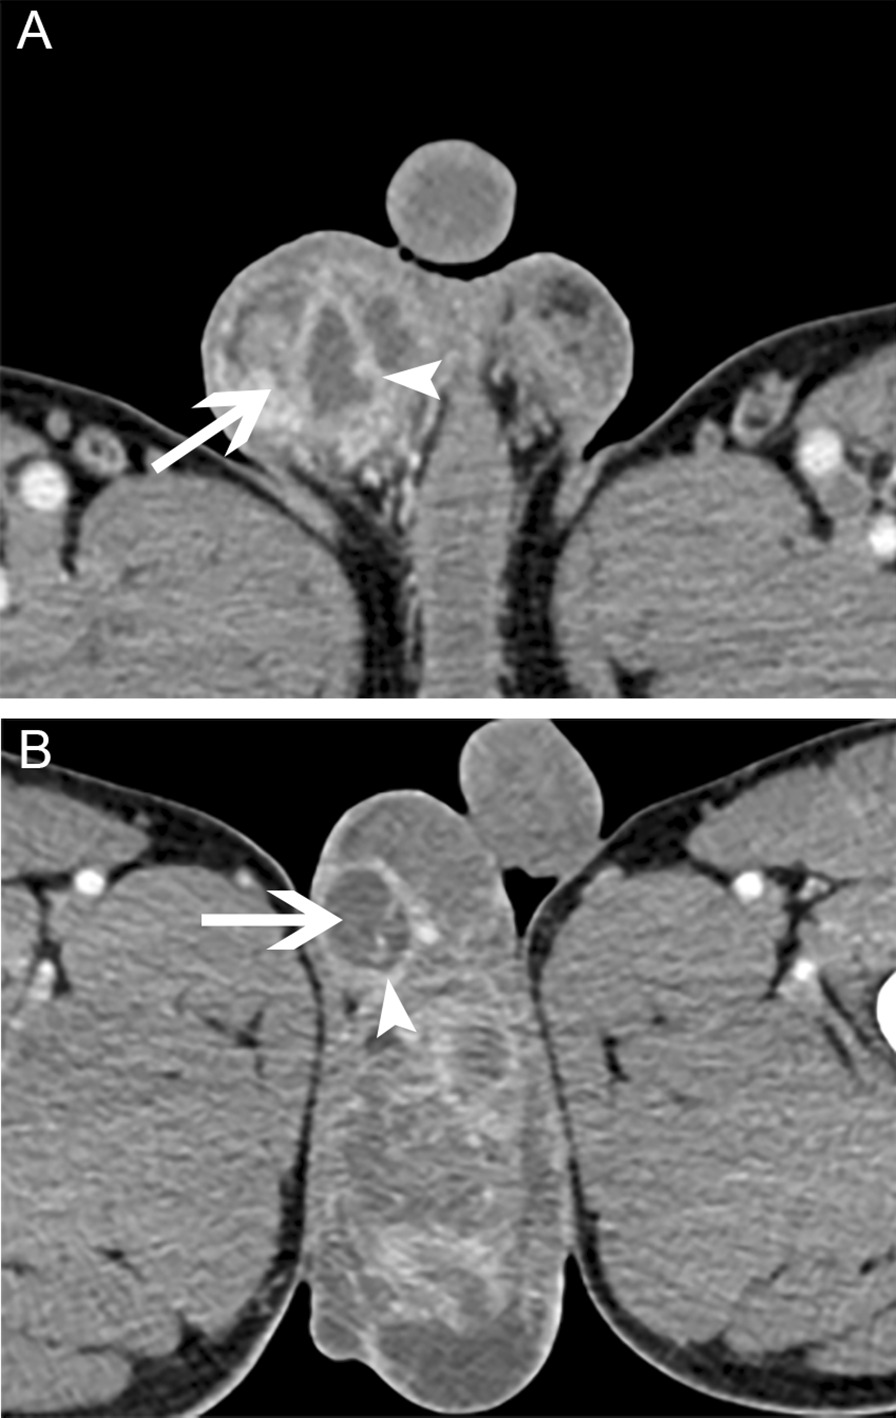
Scrotal Contrast-enhanced CT Scan Images. A contrast-enhanced CT scan showed asymmetric enlargement of the scrotum, in which the irregular mass or nodules (A, arrow), cystic lesions (B, arrow) and heterogeneous or annular enhancement (arrowheads) were observed
